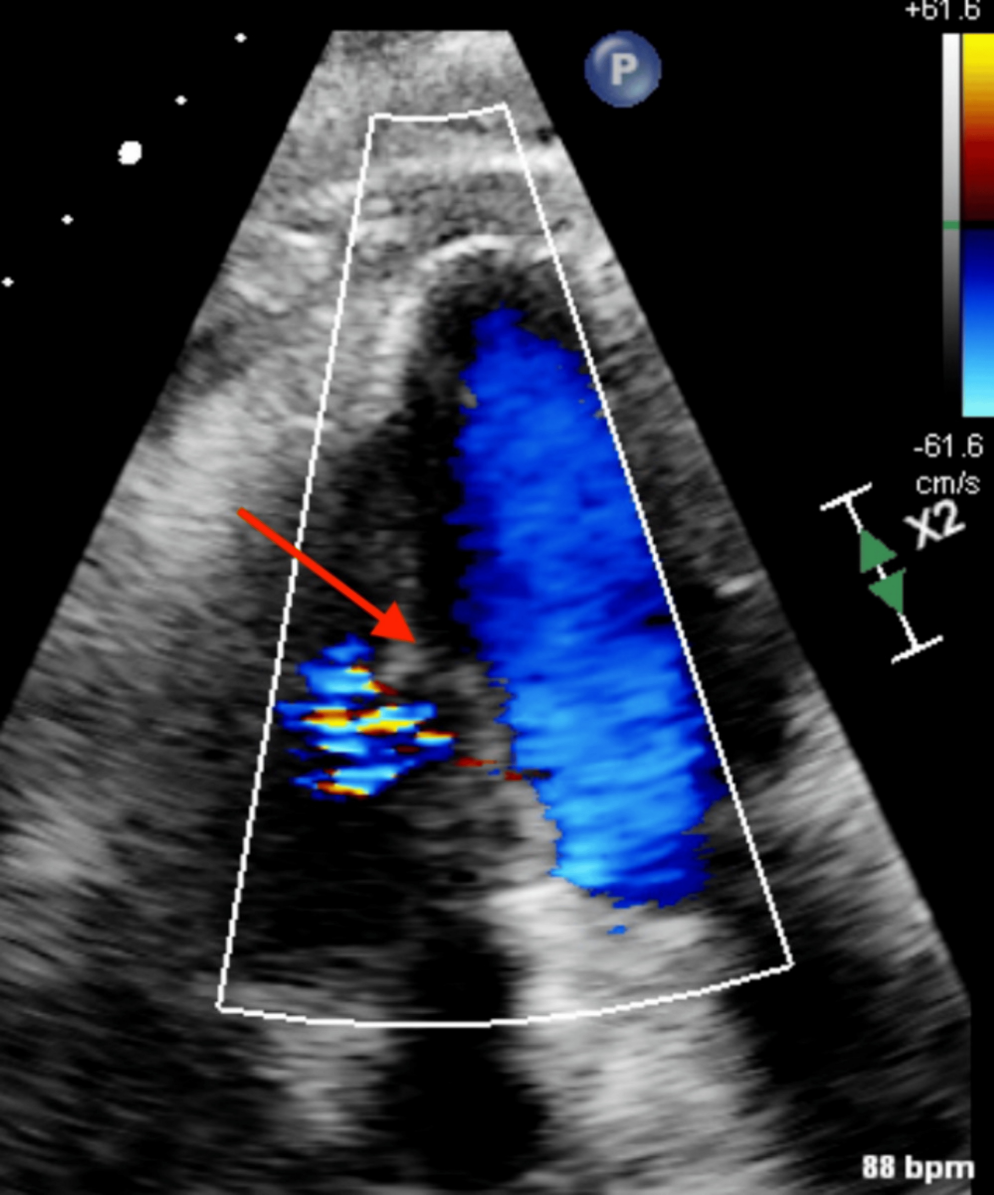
Echocardiogram done day one after the right coronary artery stent showing the ventricular septal defect
